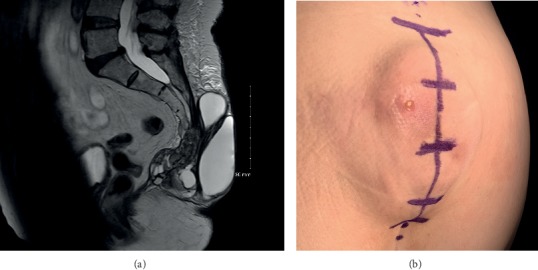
(a) T2 MRI demonstrating a large cystic lesion with a fibrous mass connected to the coccyx. (b) Intraoperative photo demonstrating large mass prior to surgical incision.
